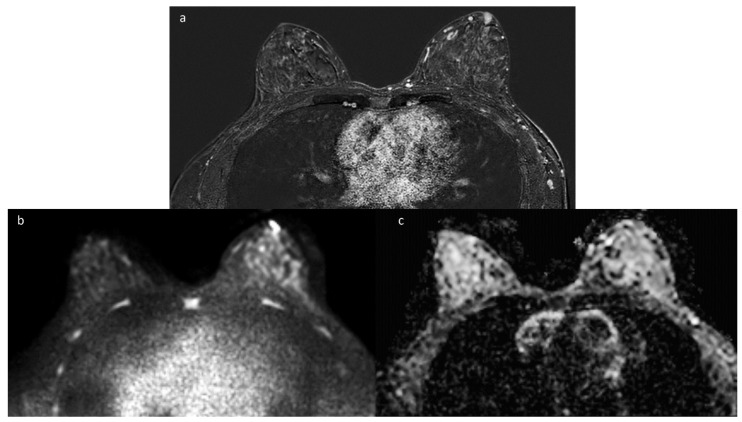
MRI subtraction image (a) of a 35-year-old woman with a DMP diagnosis following a core needle biopsy shows a heterogeneous mass-like enhancement with an oval shape in the superficial retroareolar areas of the left breast. Hyperintensity on DWI sequences (b) and signal restriction in an ADC map (c) mimic BC.
